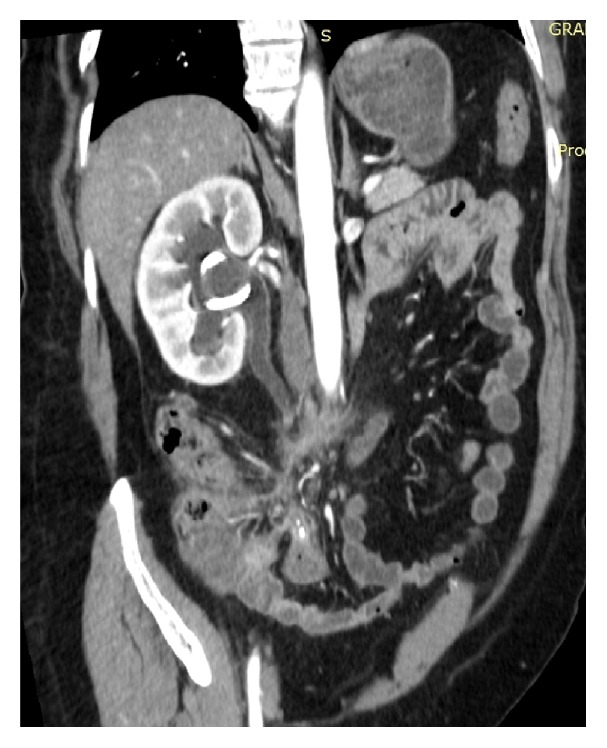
Acute appendicitis and periappendiceal inflammation obstructing right ureter on abdominal CT, oblique plane.
